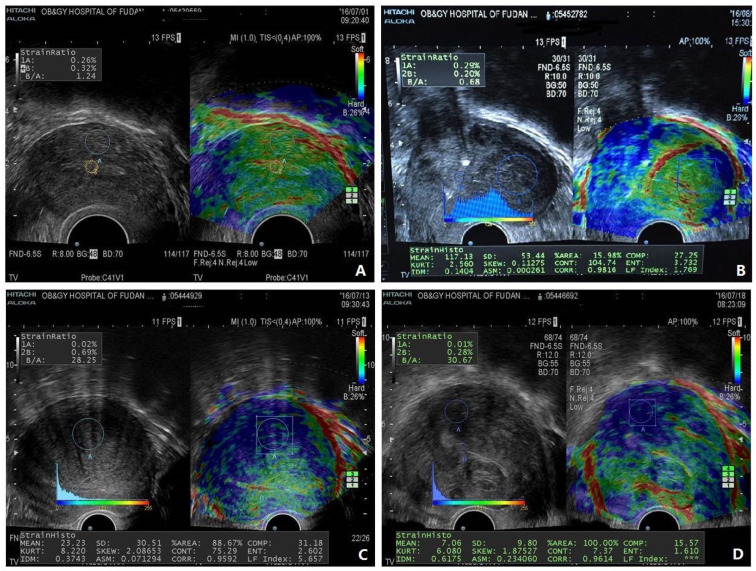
Ultrasonic strain elastographic pictures showing a normal uterus (A), uterine fibroids (B), diffuse adenomyosis (C), and focal adenomyosis (D). In (A), the normal myometrium shows the greenest and the less yellow color represents mild stiffness of the tissue. In (B), the uterine fibroid shows the greenest and the less blue color represents medium stiffness of the tissue, and there is also a typical red color pseudocapsule circling around the fibroid. In (C), diffuse adenomyotic tissue shows the broad area of blue color representing the even stiffer tissue. In (D), focal adenomyosis with co-occurrence of uterine fibroid can be seen. Focal adenomyotic tissue (the upper left area) shows the local area of blue color representing the even stiffer tissue. The uterine fibroids (the middle lower area) show a typical red-colored pseudocapsule circling around the fibroid. Typical serosal surface of uterus shown in red color could be seen in every uterus. The color bar shown on the upper right corner in each figure is the color key, showing that the tissues with the red color are the softest while those with the blue, the hardest or most rigid. The cycles indicate the region of interest (ROI), and various parameters are shown at the bottom of the figure, which are combined with the LF index, where the stiffness index, originally designed for gauge liver function (thus, “LF”), in this case represents the relative stiffness. It can be seen that both the B-mode image and the elastographic image are displayed side by side. Abbreviations used: AM: adenomyosis; Normal: normal uterus. Replicated from Liu et al. [71] (Reprinted with permission from Reproductive Sciences).
